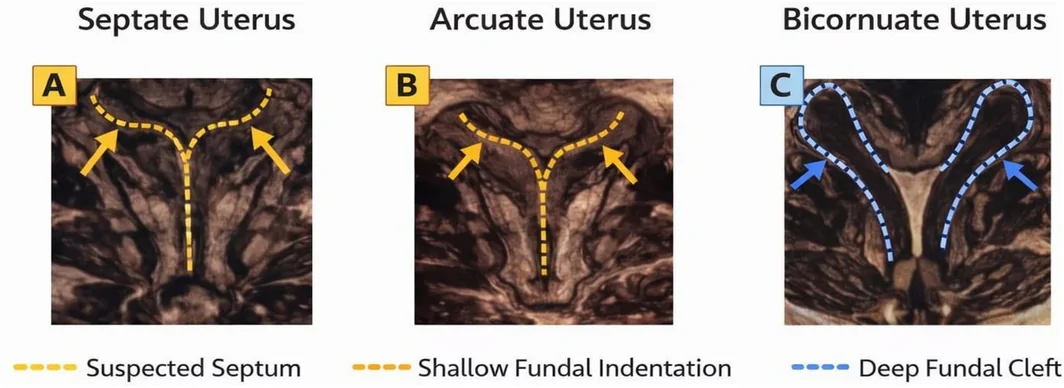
Representative T2‐weighted MRI appearances of septate, arcuate, and bicornuate uteri (educational images; NOT from the index patient). (Panel A) Coronal T2‐weighted MRI of a septate uterus, showing a linear midline indentation extending toward the endometrial cavity (yellow dashed line and arrows). (Panel B) Coronal T2‐weighted MRI of an arcuate uterus, showing a shallow concave indentation of the endometrial fundus (orange dashed line and arrows) without extension into the cavity. (Panel C) Coronal T2‐weighted MRI of a bicornuate uterus, showing a deep fundal cleft with divergent uterine horns (blue dashed line and arrows). Important note on provenance: (Panels A–C) are educational examples and do NOT correspond to the index patient, whose MRI is shown separately in Figure 2. They were obtained from copyright‐free/open‐access resources permitting reuse for non‐commercial scientific publication, with attribution per the source license.
